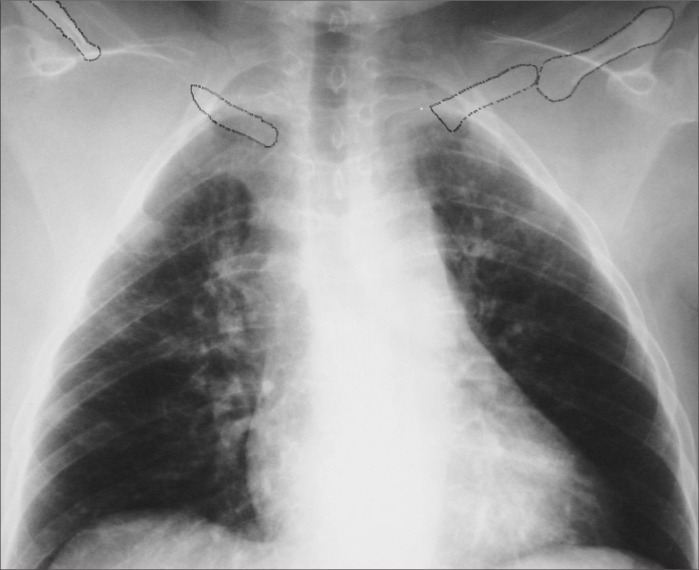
Posteroanterior view of chest radiograph showing the position of clavicular fragments in a woman with cleidocranial dysostosis.
